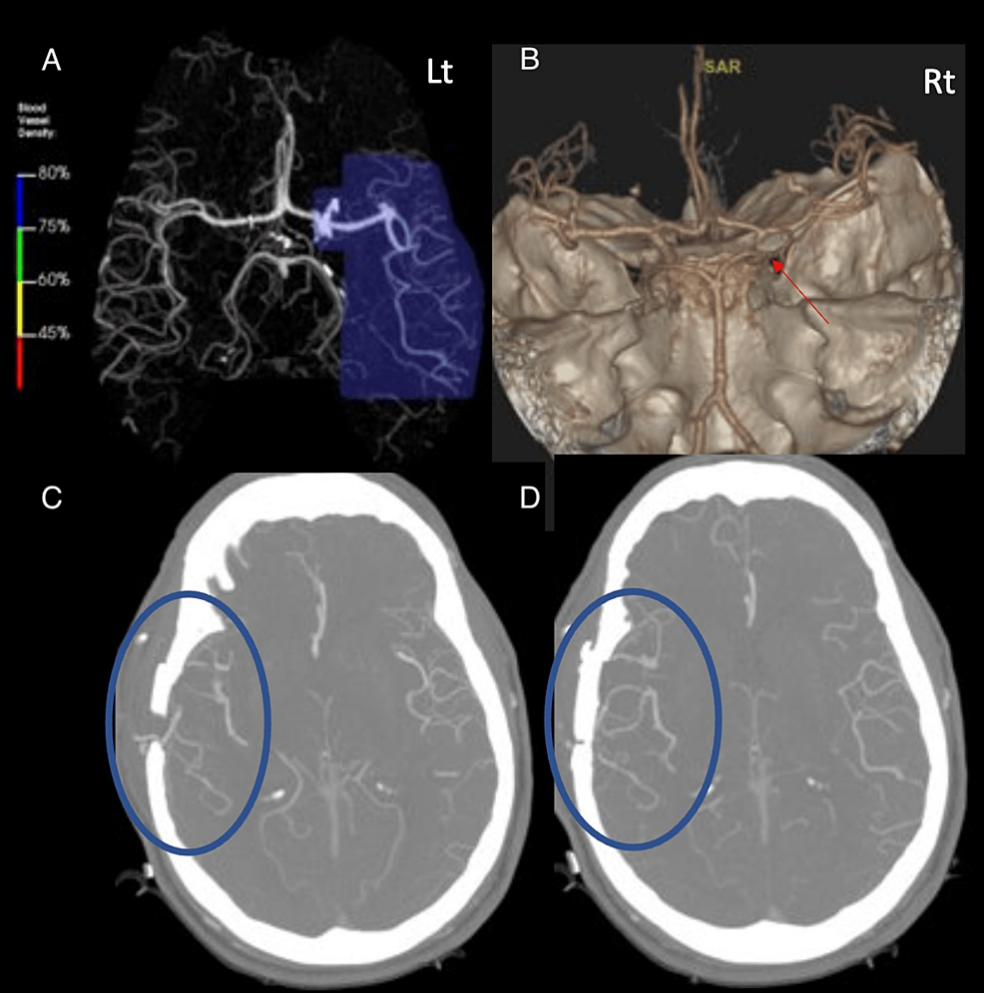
A non-contrast head CT angiogram demonstrating an STA-MCA bypass. Panels A and B depict computerized reconstruction. Panels C and D highlight an area of decreased blood vessel density due to artifact (blue circles). STA: superficial temporal artery, MCA: middle cerebral artery
